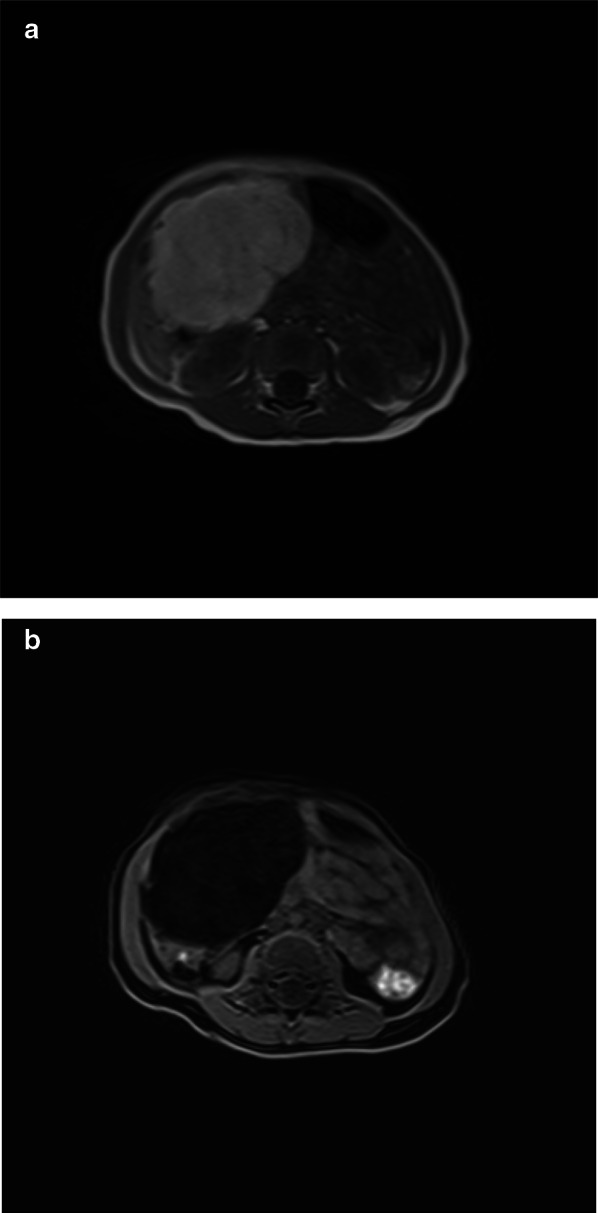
Magnetic resonance imaging coronal section image obtained a well-encapsulated soft-tissue mass lesion with high intensity in the mesenteric region, a T1-weighted image obtained the mass lesion with high intensity signal. b Fat suppression inversion recovery imaging obtained an interposing fat component showing a reduced signal
